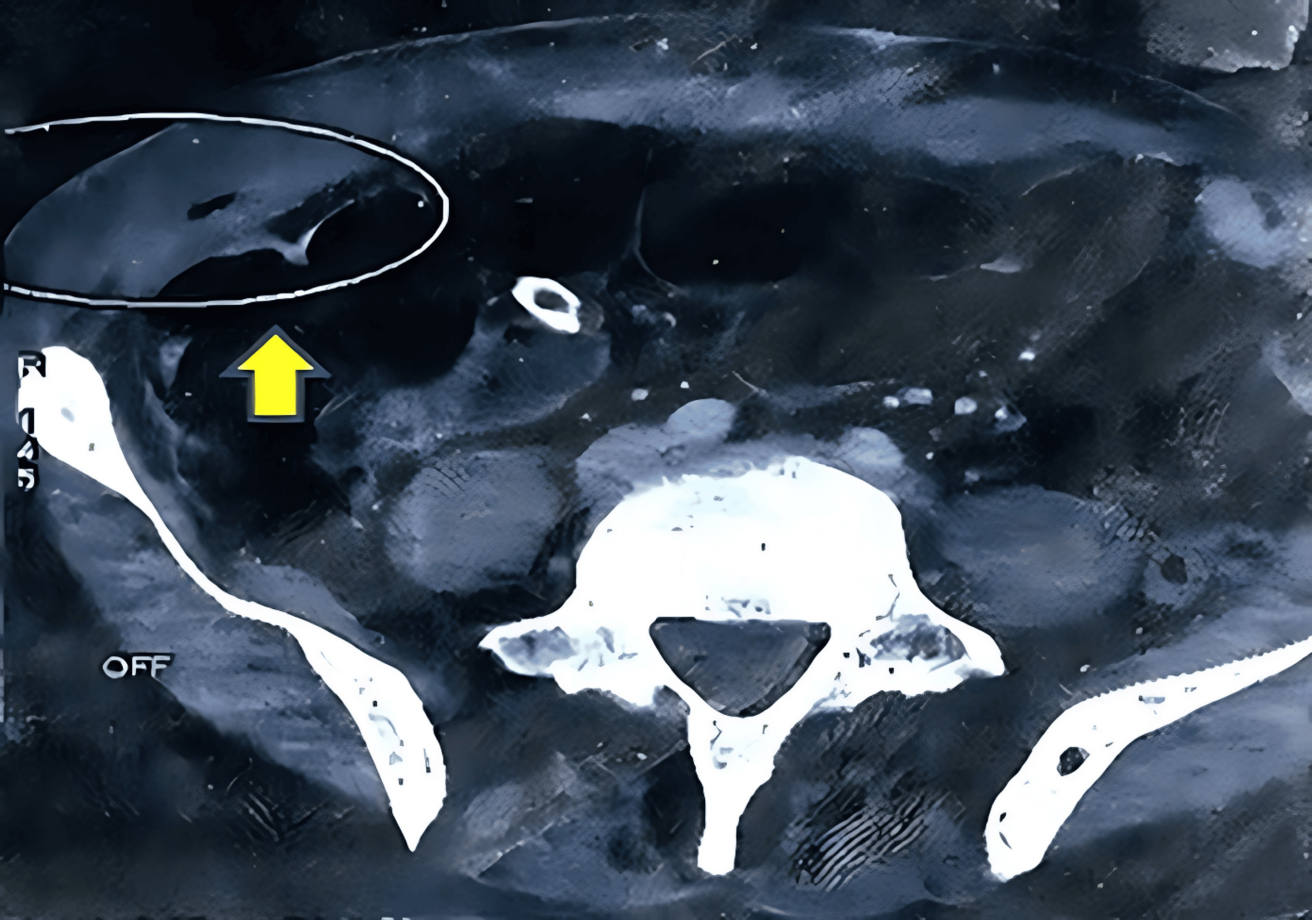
Axial CT scan section showing a right-sided parietal wall abscess with localized peritoneal reaction. Rim-enhancing fluid collection in the right parietal wall (yellow arrow, circled) is consistent with an abscess. Surrounding soft-tissue stranding indicates a localized inflammatory reaction.
